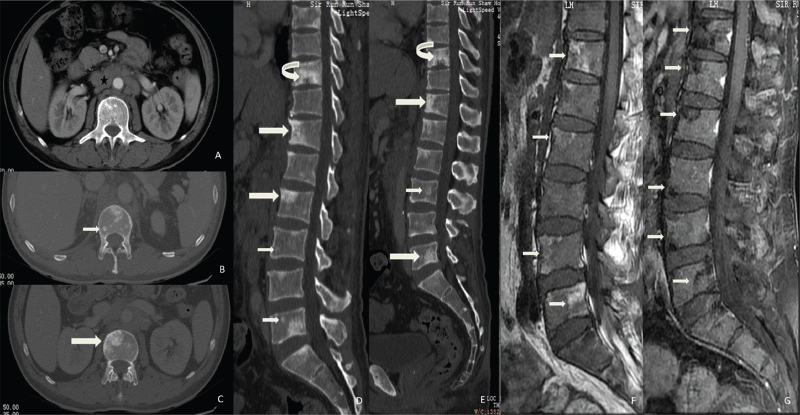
(A) Enlargement of multiple retroperitoneal lymph nodes can be seen (black star). (B-E) Multiple sclerotic lesions can be seen in the vertebral bodies: some are small ring-like high-density lesions (thin straight arrow), some are agglomerated high-density lesions with an ill-defined margin (thick straight arrow), and some appear to erode endplates (curved arrow). (F) After 5.5 months of treatment, T1-weighted imaging showed lesions gradually changing into yellow marrow, which suggested improvement (thin straight arrow). (G) After continued treatment for a further 3 months, fat saturation T1-weighted imaging with contrast showed that the lesions with yellow marrow were suppressed and did not enhance, which indicated recovery (thin straight arrow).
